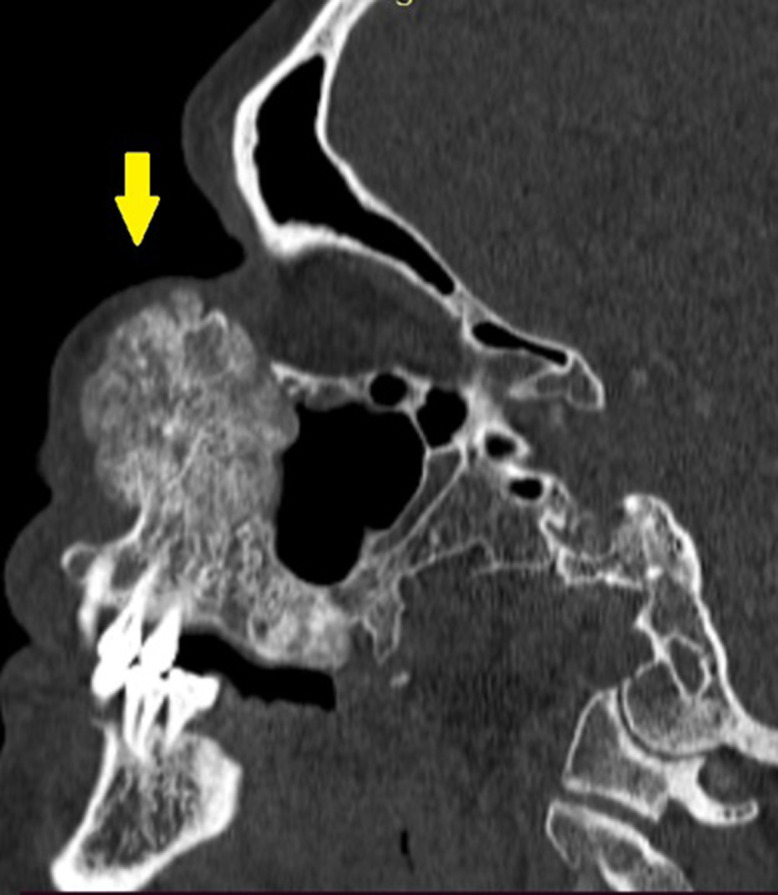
sagittal CT scans, viewed at wide window settings showing an intraosseous hemangioma arising from the left maxilla (yellow arrow)
